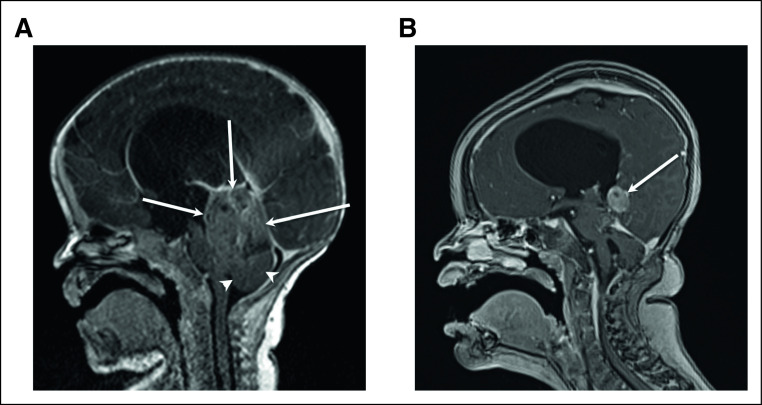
Magnetic resonance imaging of the tumor. (A) Sagittal contrast enhanced T1-weighted image at presentation at age 2 months demonstrated a large avidly enhancing mass situated in the quadrigeminal plate cistern of the posterior fossa (arrows), with superior displacement of the tentorium and straight sinus and inferior displacement of the cerebellar vermis (arrowheads). (B) Sagittal contrast enhanced T1-weighted image at relapse at age 3.5 years demonstrated a recurrent tumoral nodule with avid contrast enhancement, similar to the primary tumor.
